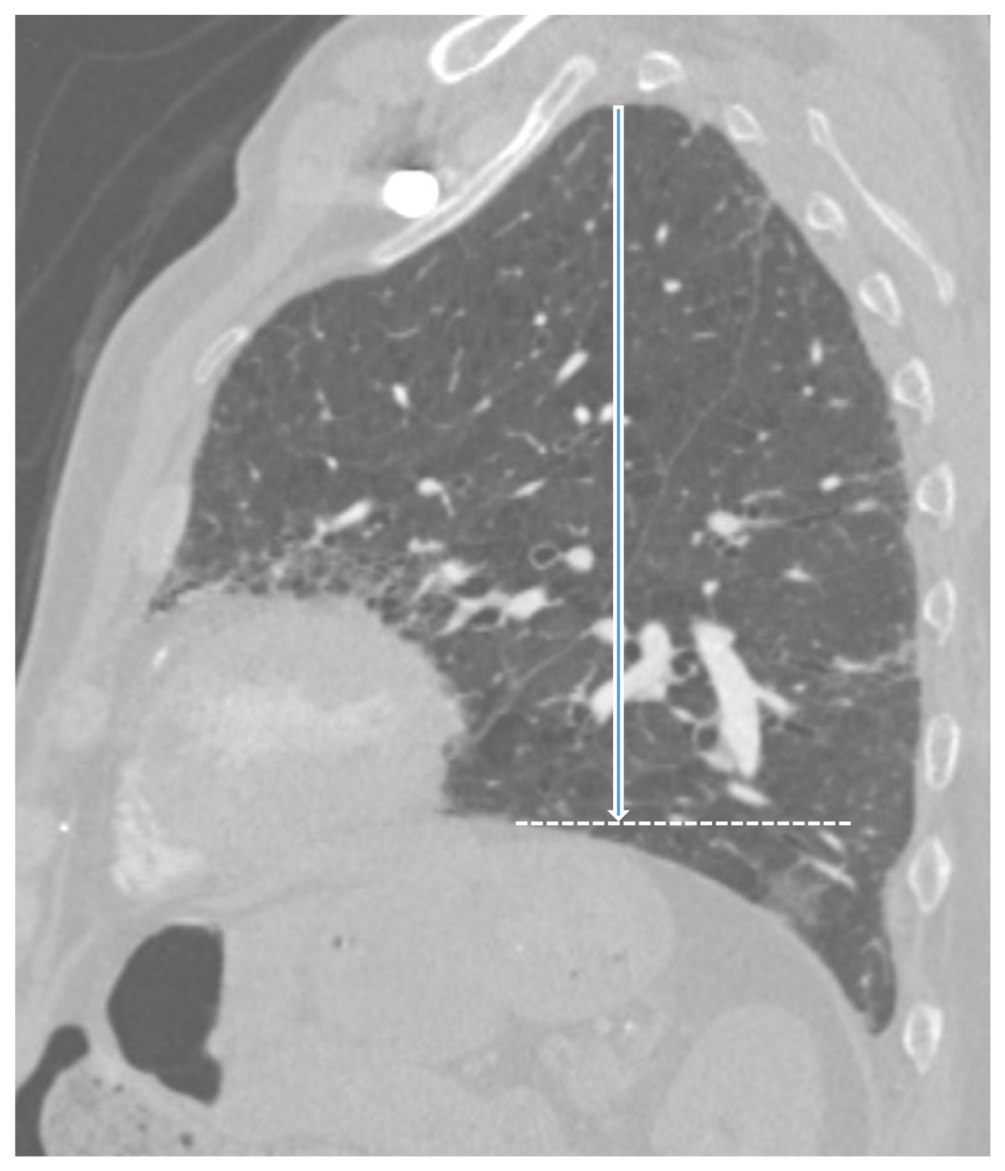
Sagittal contrast enhanced CT image of an 83-year-old male with IPF. CT image shows lung height (white arrow), drawn from lung apex to the dome of diaphragm (dashed line) at midclavicular line. Lung height was corrected for body habitus by dividing it by sagittal height of the tallest vertebrae in lower third of thoracic spine (not shown).
